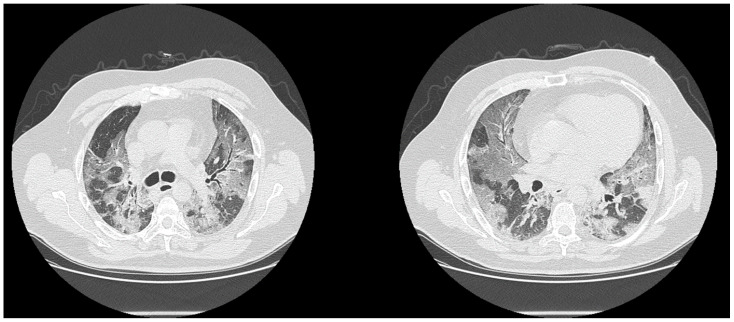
The CT images show a typical aspect of bilateral extended crazy paving characterized by GGO with septal thickening, patchy distribution, and aerial bronchogram.
